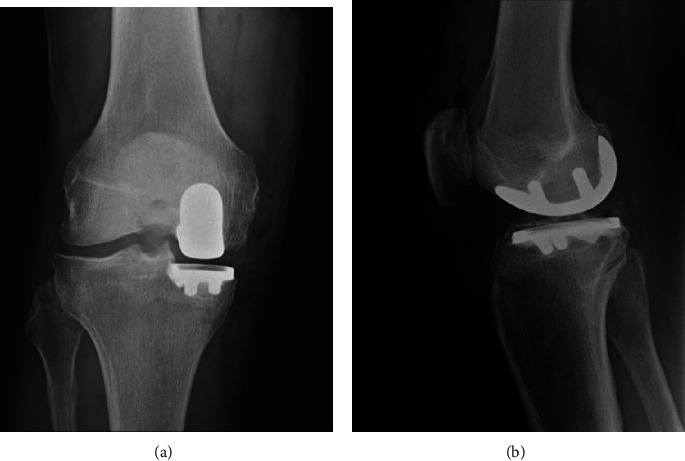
Unicompartmental knee arthroplasty in anatomic alignment. (a) Anteroposterior radiograph. (b) Lateral radiograph.
